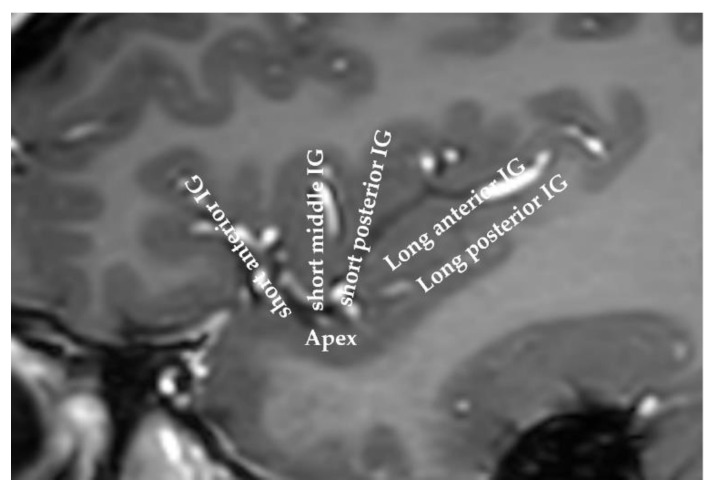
MRI of a human insula. The anatomy of the insula can be viewed on this sagittal slice. The insula is classically divided into the anterior insula and the posterior insula, which are separated by the central insular sulcus. IG: insular gyrus.
